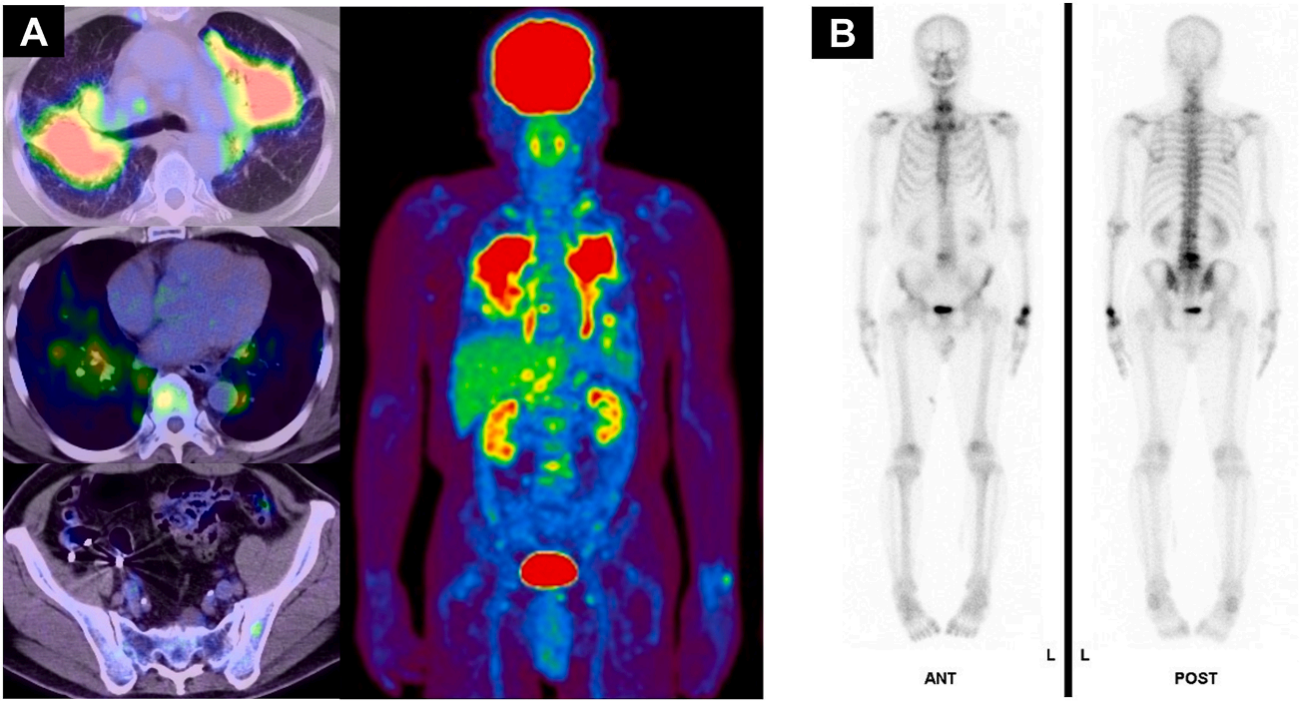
(A) Pretreatment FDG-PET/CT demonstrated multiple areas of uptake in the lungs, lymph nodes (cervical, supraclavicular, mediastinal, hilar, paraaortic, iliac, and inguinal lymph nodes), and multiple bones (thoracic vertebrae, lumbar vertebrae, and pelvic bone). (B) Pretreatment bone scintigraphy demonstrated uptake in the lumbar vertebrae and left carpal bone.
